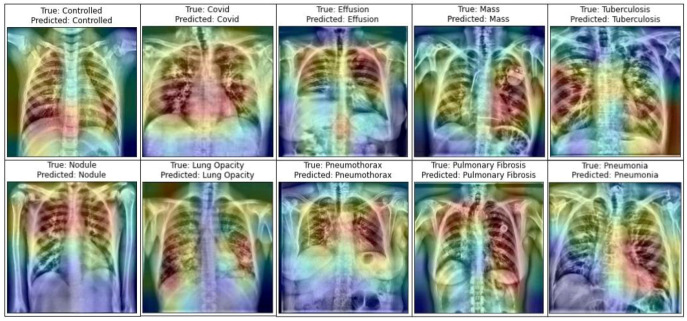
Representation of infected area of lung in X-ray images utilizing Grad-CAM on the LungNet22 model.
